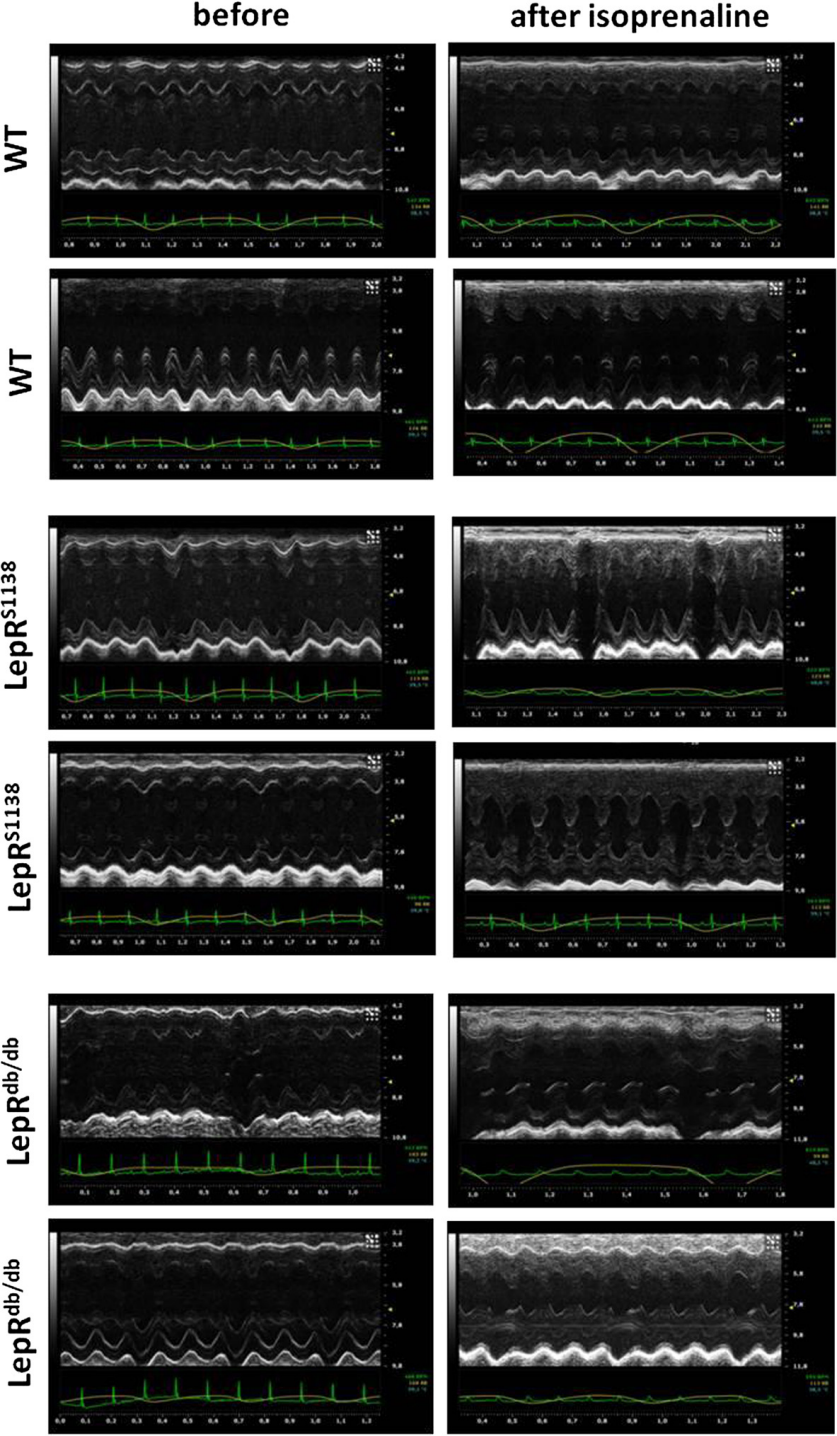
Representative M-mode echocardiography recordings.
